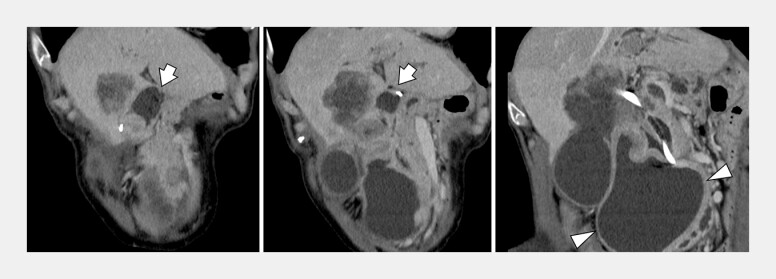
Computed tomography shows a tensed end loop (arrow) and expansion of the submucosal space in the horizontal part of the duodenum (arrowhead).
